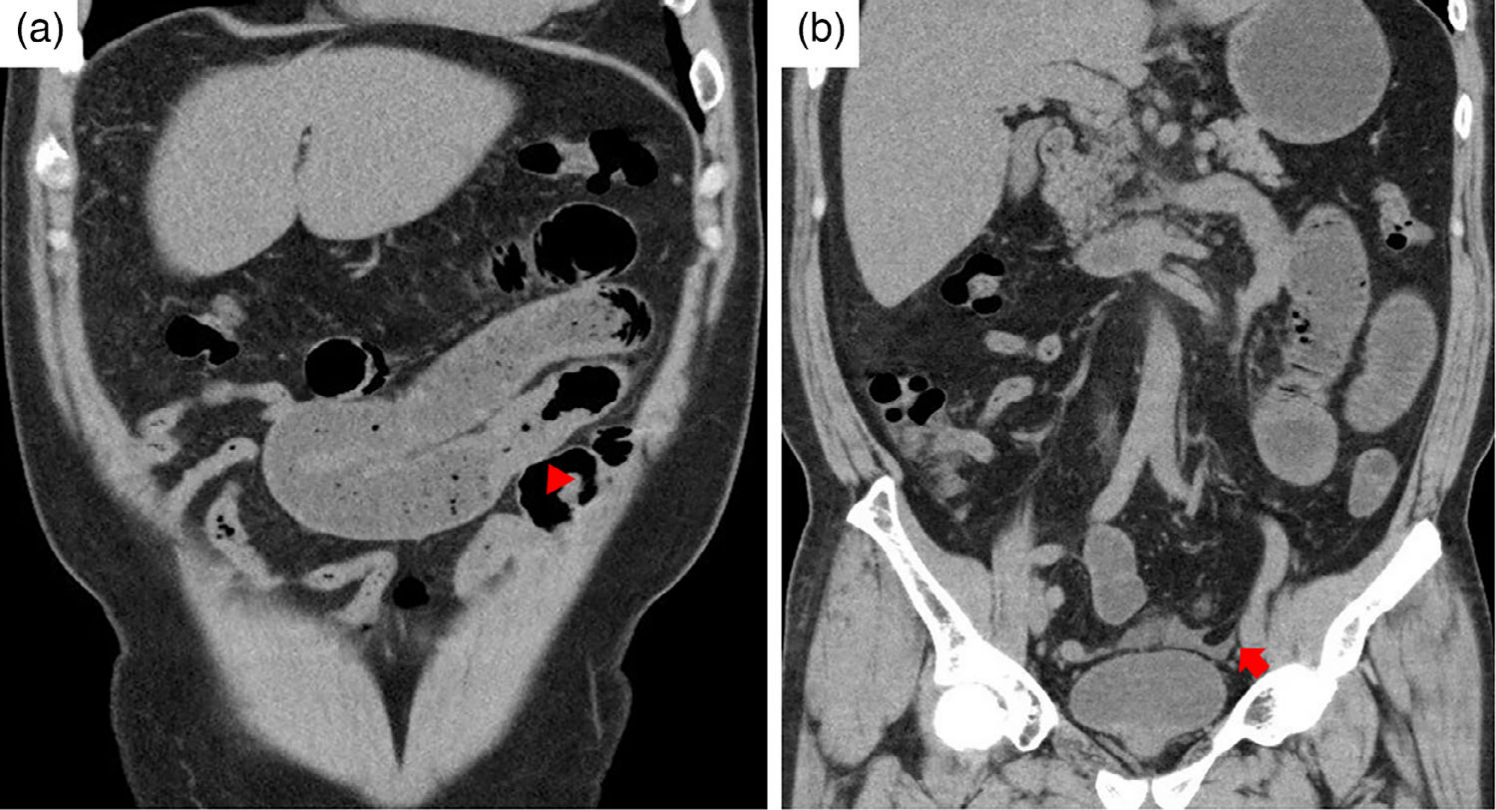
Plain‐computed tomography scan on the day of admission showed edematous wall thickening of the jejunum (red triangle), dilation of the proximal side (a), and a small amount of ascites (red arrow) (b).
